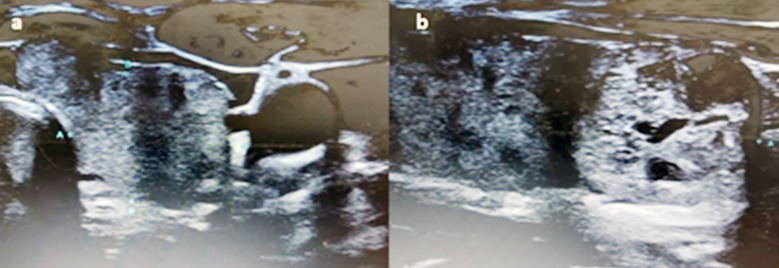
Pre-operative ultrasonography showing the left thyroid lobe on axial view (a) and craniocaudal view (b). No suspicious parathyroid gland detected.
